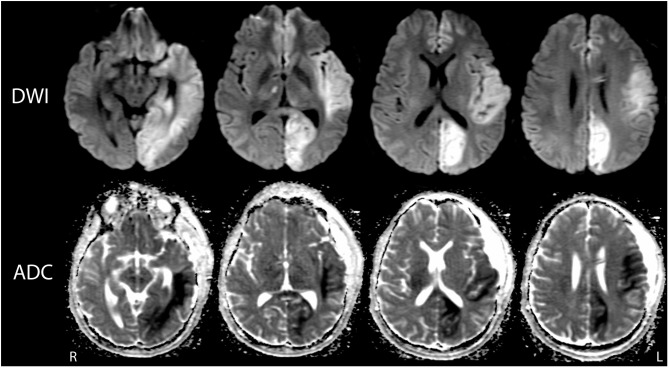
Multifocal infarctions due to vascular compression during transtentorial herniation. Representative diffusion-weighted images (DWI; top row) and apparent diffusion coefficient (ADC; bottom row) images demonstrate ischemic infarctions with the anterior cerebral artery, middle cerebral artery, and posterior cerebral artery territories. The infarctions are indicated by hyperintense signal on DWI and corresponding hypointense signal on ADC. At the time of this MRI scan (post-herniation day 6), the patient's behavioral assessment indicated a minimally conscious state.
